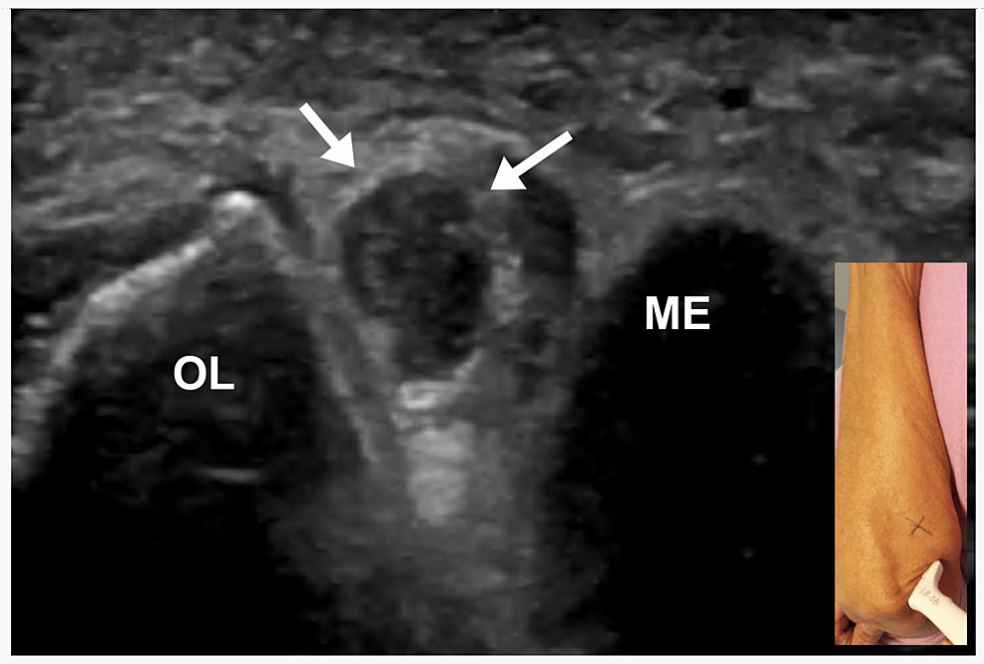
Ultrasound Study of the Ulnar Nerve at the Elbow Short-axis view of the right ulnar nerve (oblique arrows) at the elbow showing an increase in the cross-sectional area of 16 mm^2^ (normal: <10 mm^2^) ME, medial epicondyle; OL, olecranon
